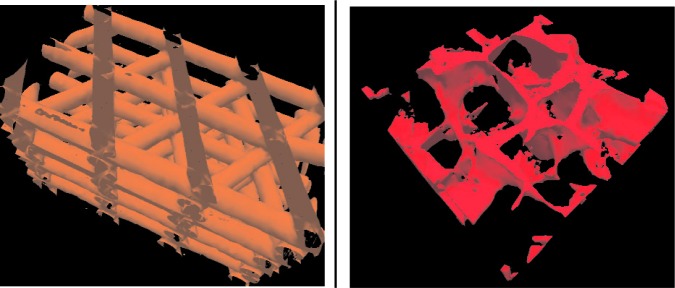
Three-dimensional representations of the X-ray μ-CAT data. for Scaffolds A and B.
